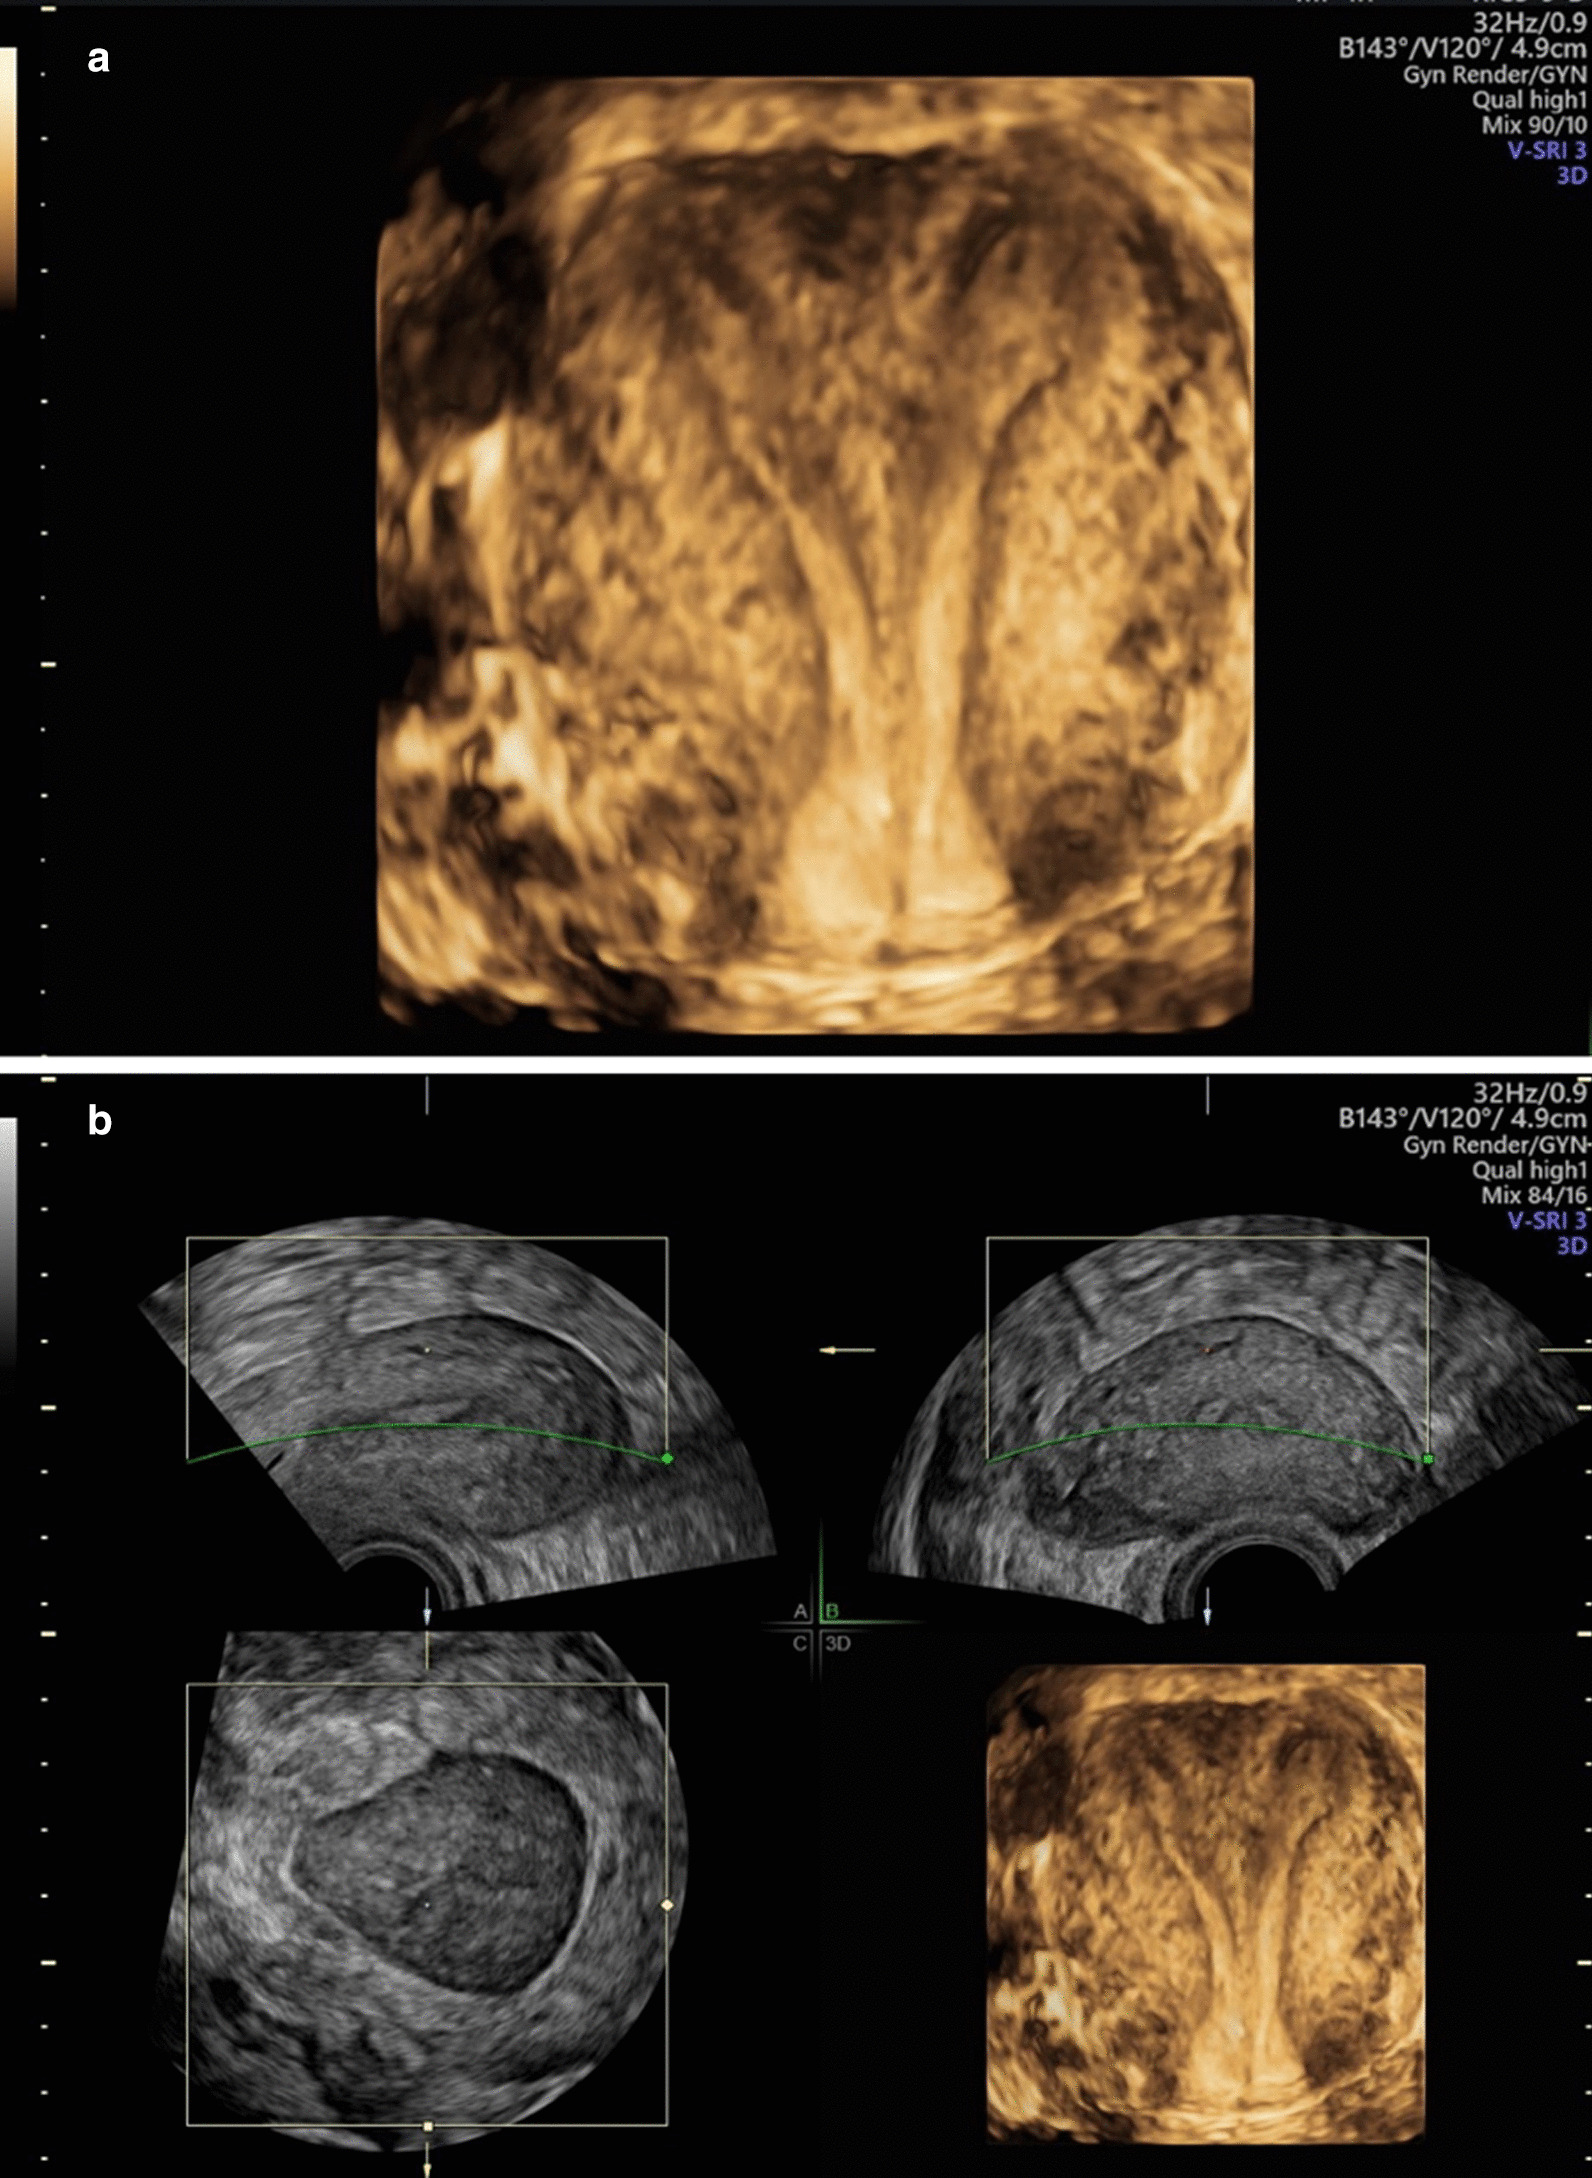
3D ultrasound at 8 weeks postpartum; a Bicornuate bicollis uterus (functional unicollis) in the transverse plane with both uterine cavities. b Bicornuate bicollis uterus (functional unicollis): cross-section through both uterine cavities, 3D ultrasound
